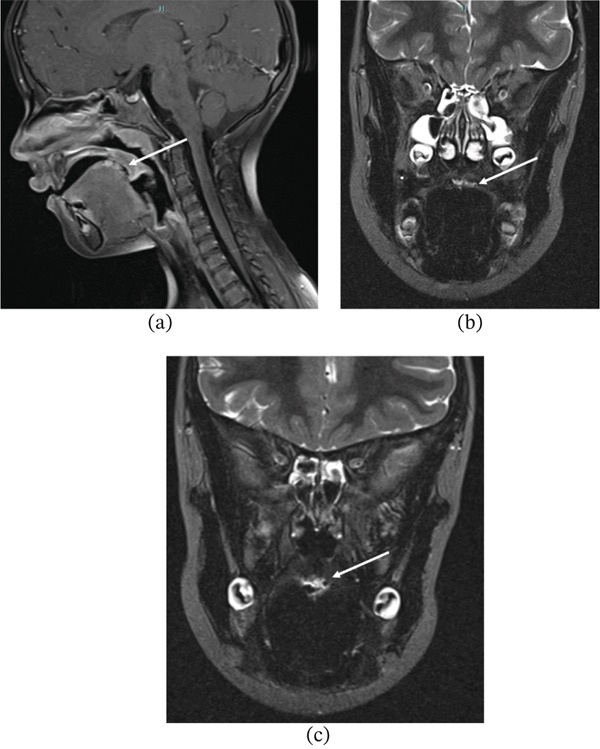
Magnetic resonance imaging of the lesion. (a) Sagittal T2‐weighted image showing a well‐defined hyperintense lesion (arrow) on the dorsal tongue. (b) Axial T2‐weighted image confirming superficial localization without deep infiltration (arrow). (c) Postcontrast T1‐weighted image with fat suppression demonstrating mild homogeneous enhancement (arrow). MRI findings were suggestive of a vascular lesion, possibly hemangioma, without definitive diagnostic features.
